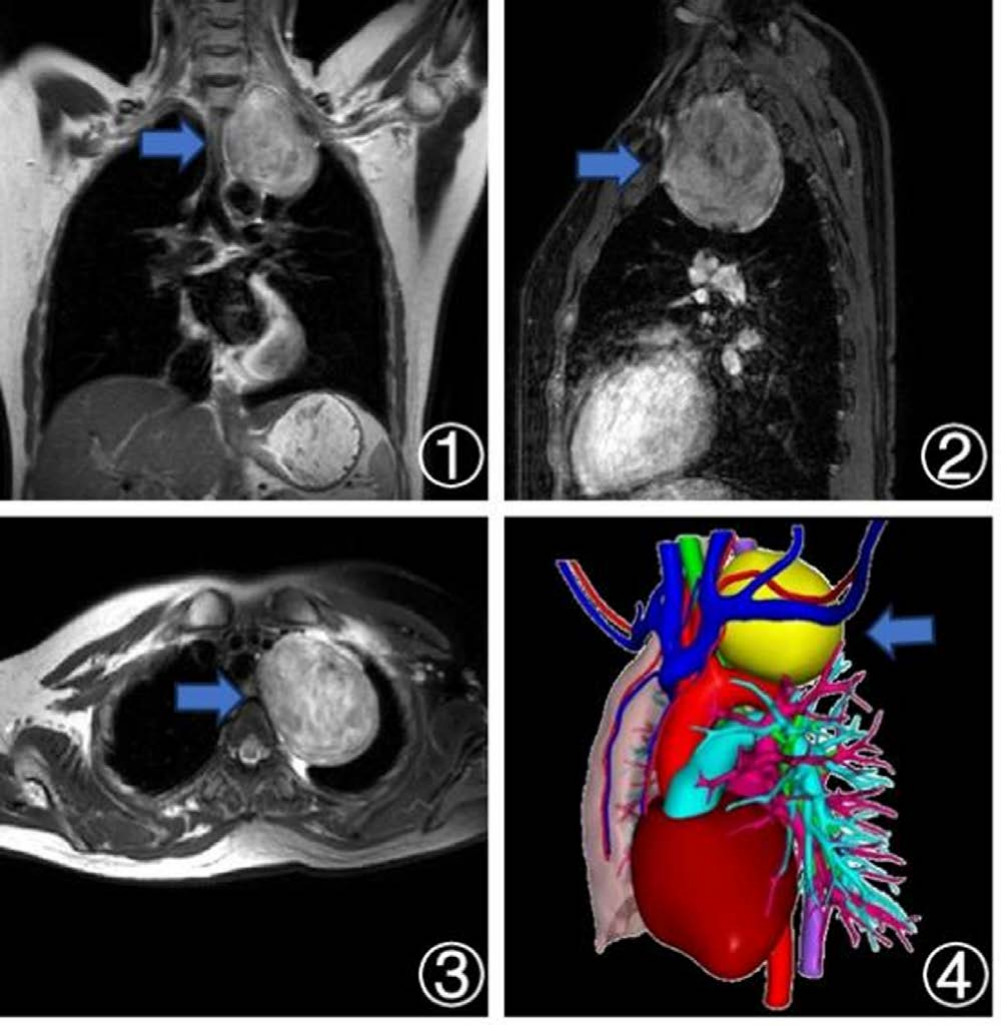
Chest magnetic resonance images and three-dimensional reconstruction model: ① coronal view; ② sagittal view; ③ axial view; ④ three-dimensional reconstruction model of the heart, major blood vessels, and tumor.
